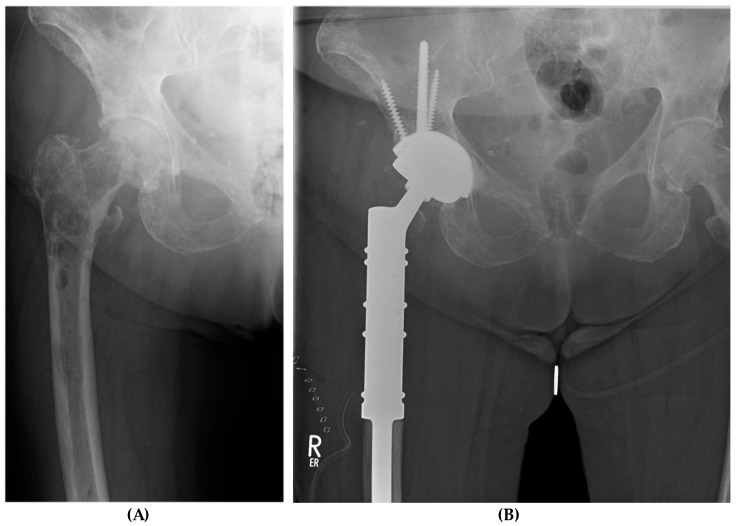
Case report of a 75-year-old female (#12); metastatic breast cancer. (A) Local progress of metastatic destruction femoral and peri-acetabular after radiation. Immobilizing and therapy-resistant pain. (B) Radiograph post-surgery; implant system: MUTARS^®^ PRS^®^ (Pelvic Revision Shell; Implantcast GmbH, Buxtehude, Germany); MUTARS^®^ Proximal Femoral Replacement (Implantcast GmbH, Buxtehude, Germany). Patient survival at 36 months, absence of pain; patient regularly uses a cane for longer walking distances.
